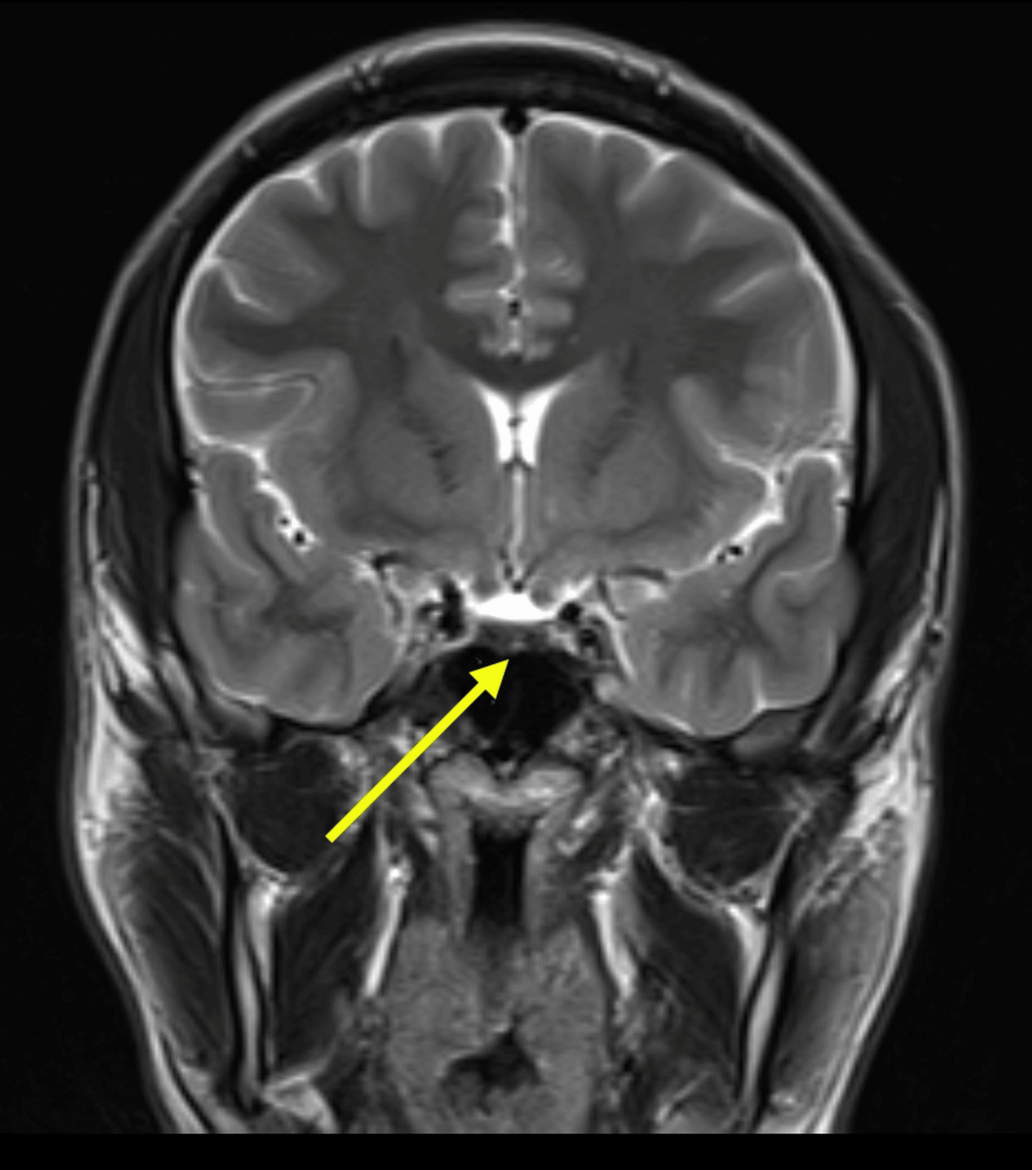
Coronal T2‑weighted MRI of the brain demonstrating the sella turcica and pituitary gland (yellow arrow) with normal size, morphology, and signal intensity. No evidence of focal lesions or suprasellar extension is seen.
